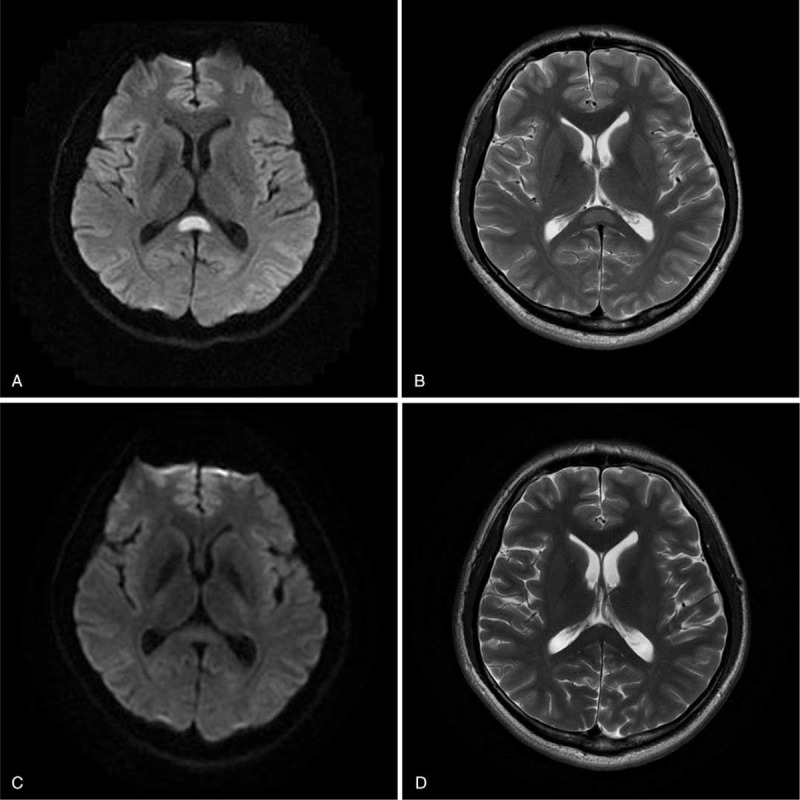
In case 3, a 29-year-old man presented with fever and headache. This patient was clinically diagnosed with tuberculous meningitis. The initial 3.0 T MRI indicated a lesion in the splenium and a hyperintensity on the DWI (A) and T2-weighted (B) images; The follow-up1.5 T MRI indicated the resolution of the splenial lesion at 15 days after the initial MRI examination (C,D).
